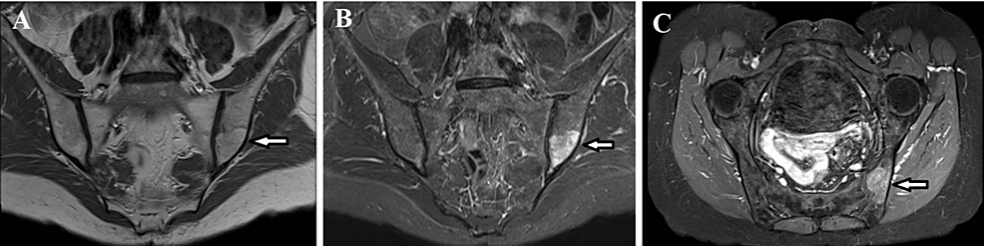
MRI imaging demonstrates the lesion in the left iliac bone (white arrows). (A) Coronal T1-weighted image shows a moderately high signal intensity, appearing brighter than the bone marrow and lower than subcutaneous fat. (B) Coronal short tau inversion recovery (STIR) sequence demonstrates a high-signal, ill-defined, and heterogeneous lesion. (C) Axial T1-weighted image with fat suppression reveals contrast enhancement after gadolinium injection.
